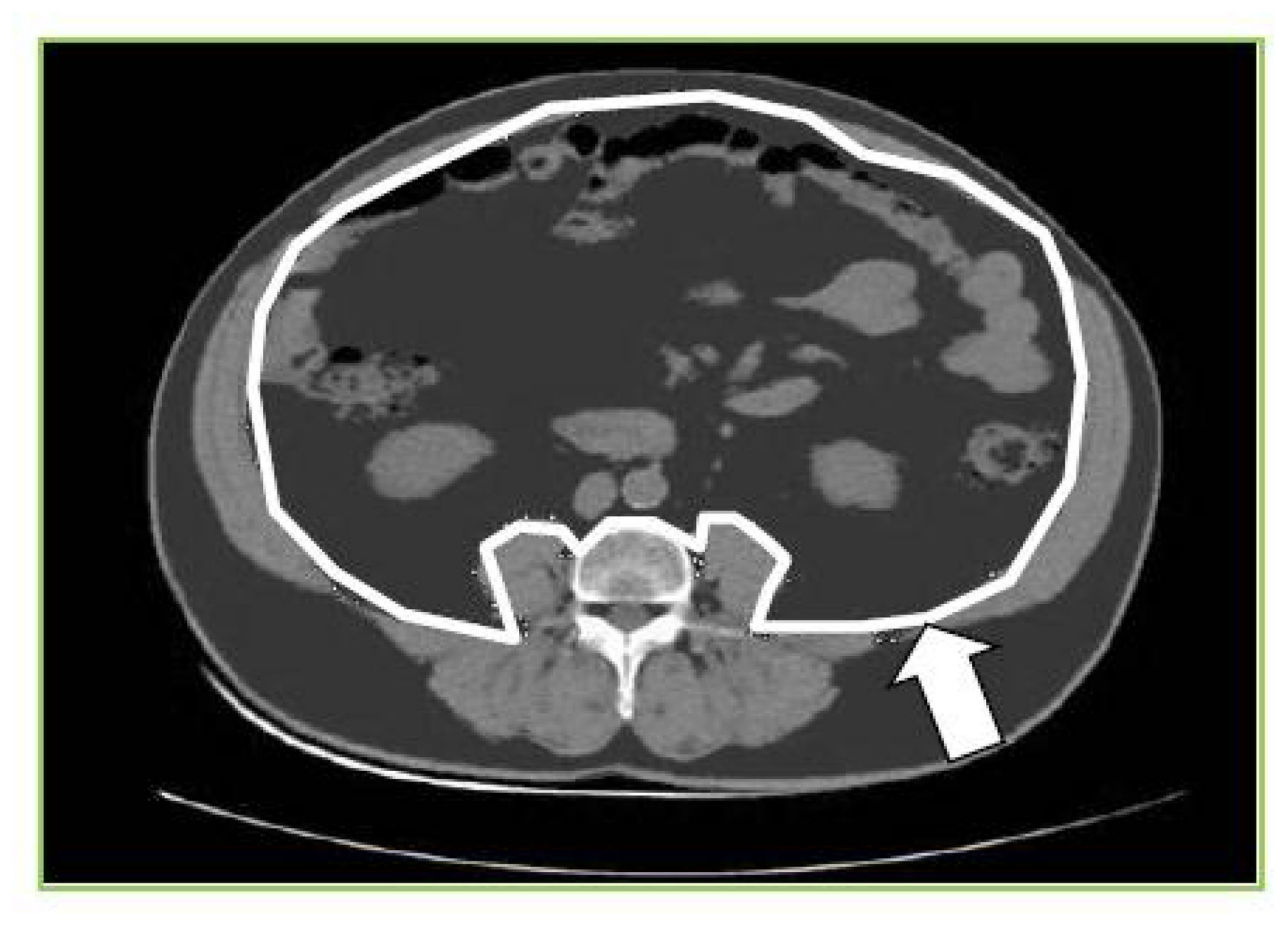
Measurement of VAT and SAT from CT image at L4. Adipose tissue is dark grey, white line shows delineation of visceral compartment, white arrow indicates depth of subcutaneous compartment.
